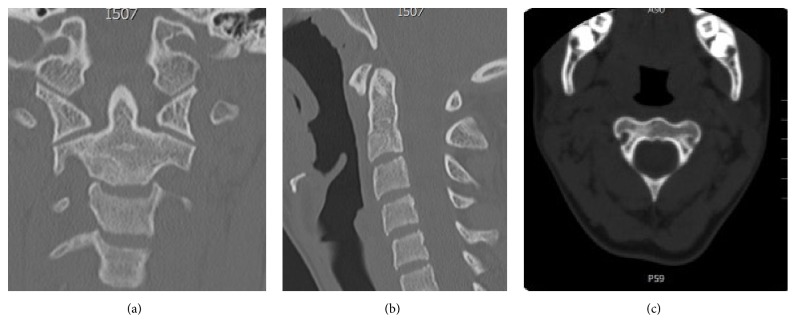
(a), (b) Postoperative CT scan that shows no recurrence and adequate integration of the graft. (c) An image corresponding to postoperative sagittal CT.
